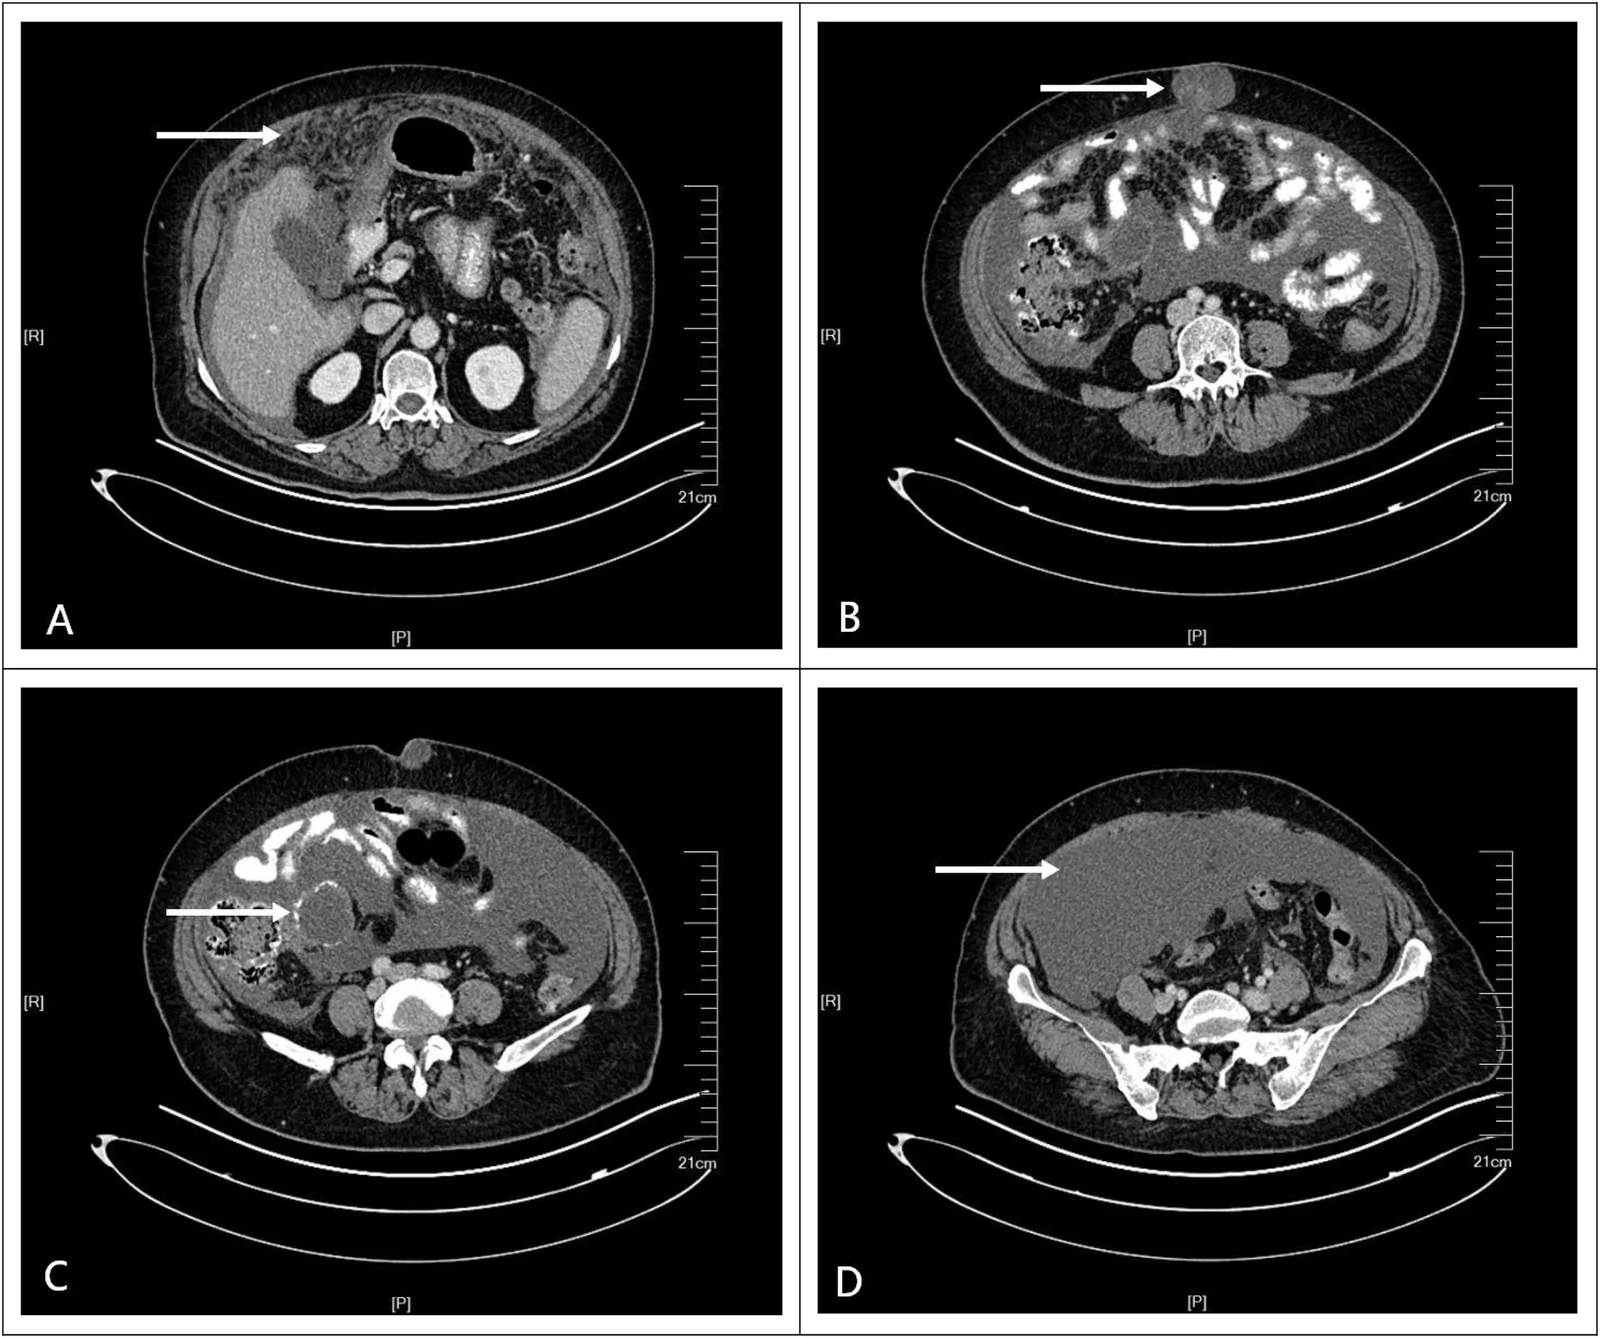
CT: (A) Thickened omentum; (B) periumbilical mass; (C) dilated and enlarged appendix in the right lower quadrant; (D) abdominopelvic effusion.
